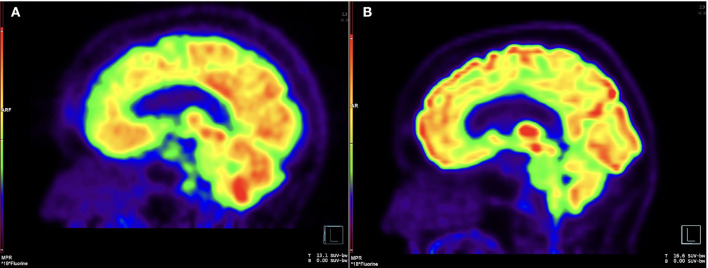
Brain FDG-PET, pre- and post-intrathecal rituximab. (A) Sagittal image from 18F-FDG PET before intrathecal rituximab demonstrates diffuse, patchy cerebral cortical hypometabolism and intense nodular uptake along the retroclival region, corresponding to areas of pachymeningeal disease. (B) Follow-up imaging after intrathecal rituximab demonstrates interval resolution of previously seen cortical hypometabolism and abnormal retroclival uptake.
